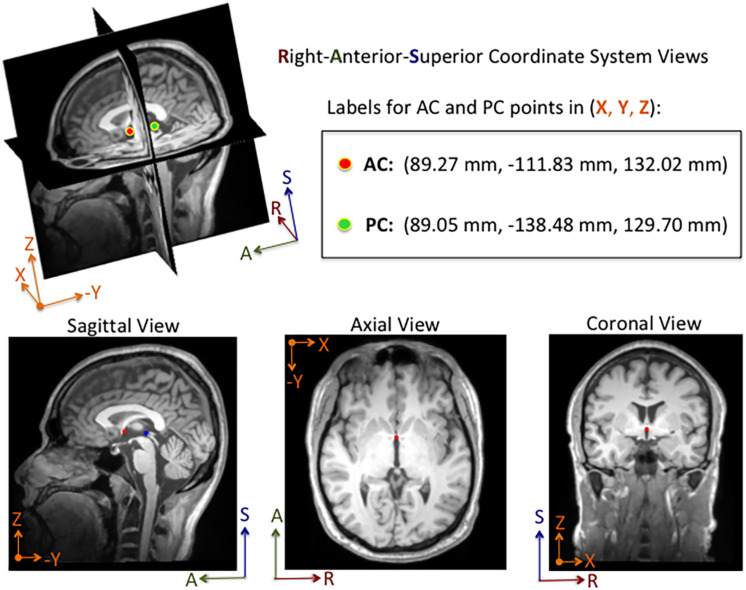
Example of an MRI volume annotated using 3D Slicer software. The volume is displayed in Right-Anterior-Superior (RAS) coordinate space. The annotator manually adjusts fiducial markers overlaid on the AC and PC points from linked 2-D views. For this subject, the AC and PC points are both visible in the same sagittal slice, whereas only the AC point is visible in the axial and coronal slices shown here. Depending on the orientation of the brain within the MRI volume, the AC and PC points are sometimes both visible within a single sagittal and an axial slice. The 3D coordinates for each point are displayed in millimeters in the Cartesian X-Y-Z space. The labels and corresponding 3D coordinates are saved to a fiducial comma-separated values (.fcsv) file.
